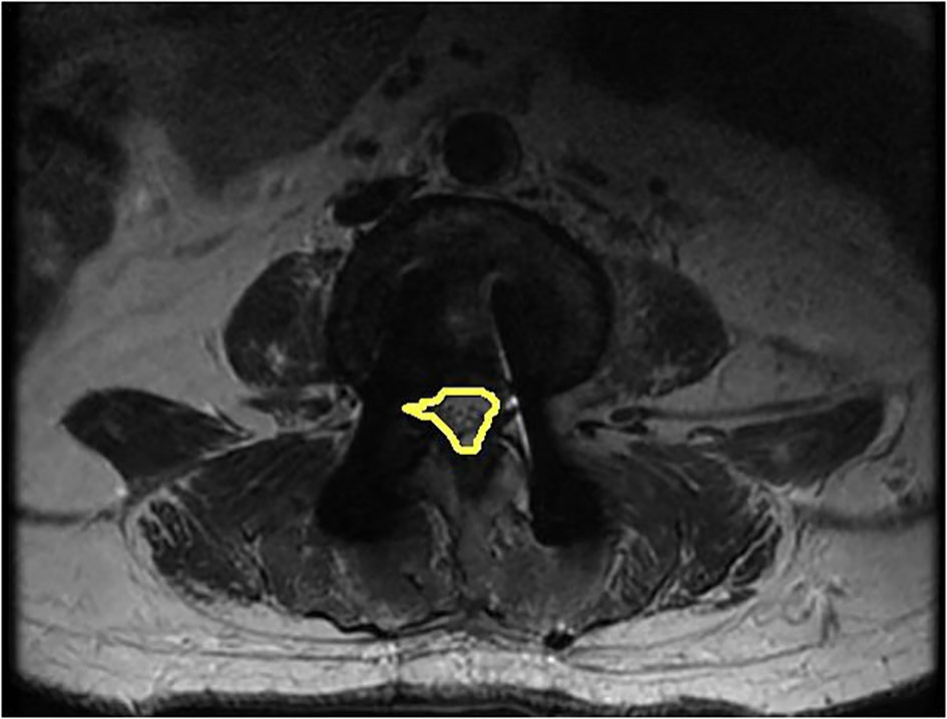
Measurement of the radiographic parameters of ASC.
